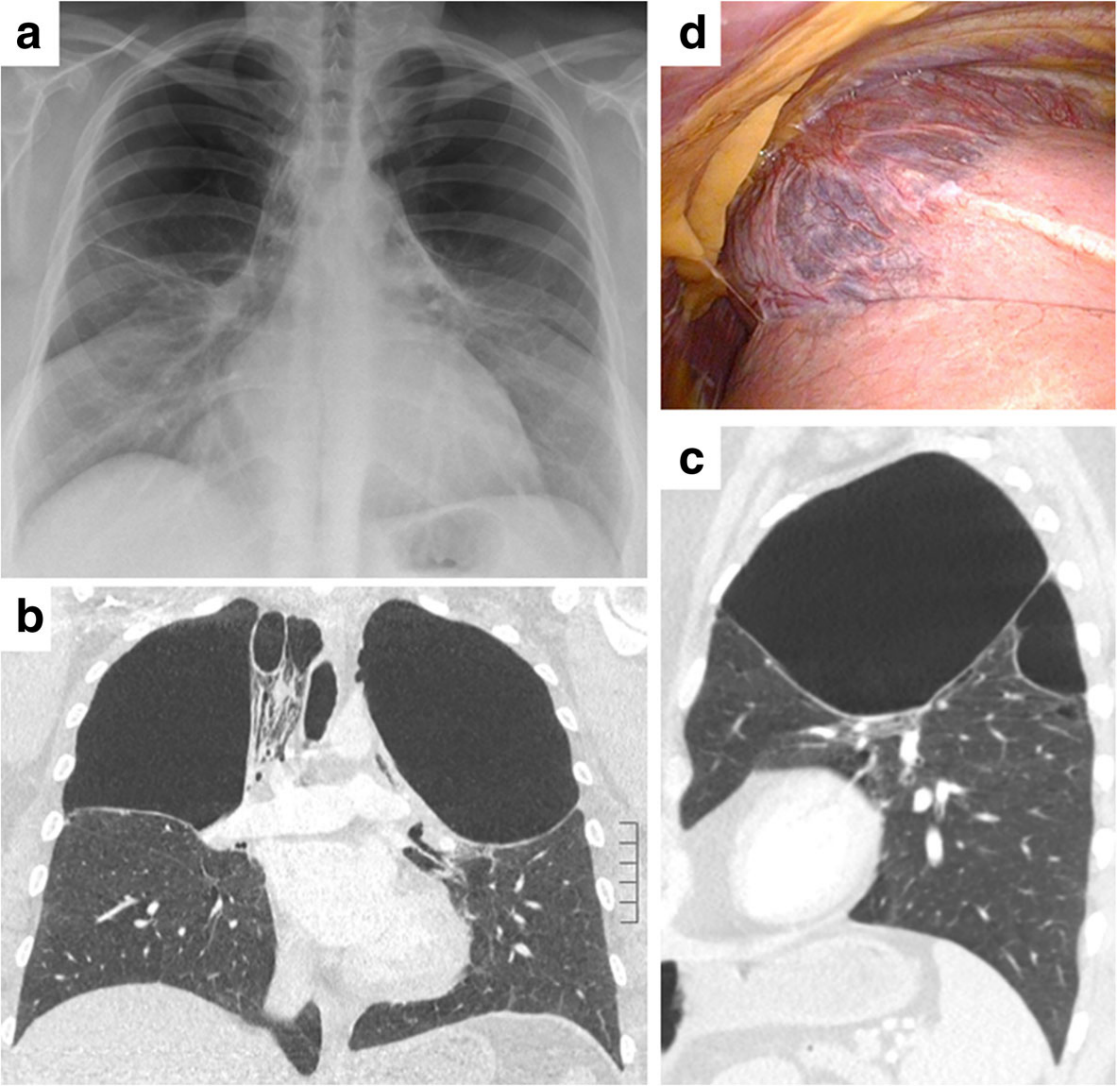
X-ray and computed tomography of the chest revealing large and symmetrical apical bullae (a-c). Intraoperatively, these bullae could be confirmed on the *right side* to originate mainly from the *upper* lung lobe (d)
